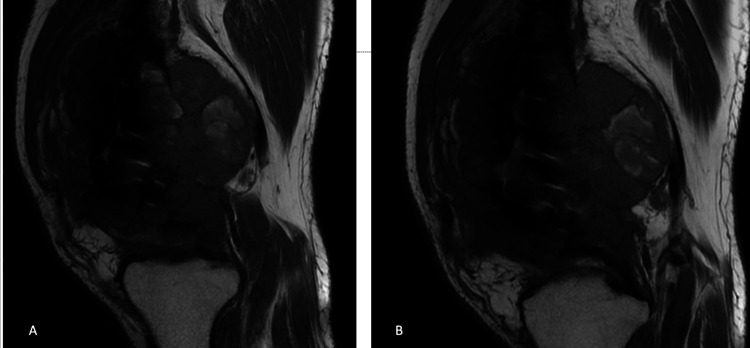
Magnetic resonance images of the patient. (A-B) Sagittal views of the patient.
